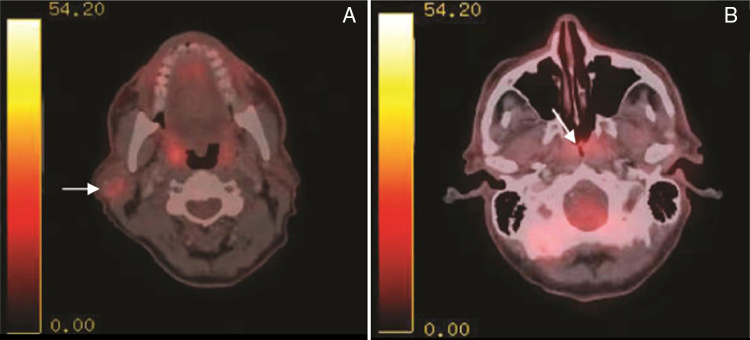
PET-CT with intravenous injection of f-18 Fludeoxyglucose (FDG). (A) White arrow – right sided parotid mass enhancement. (B) White arrow nasopharyngeal enhancement.
